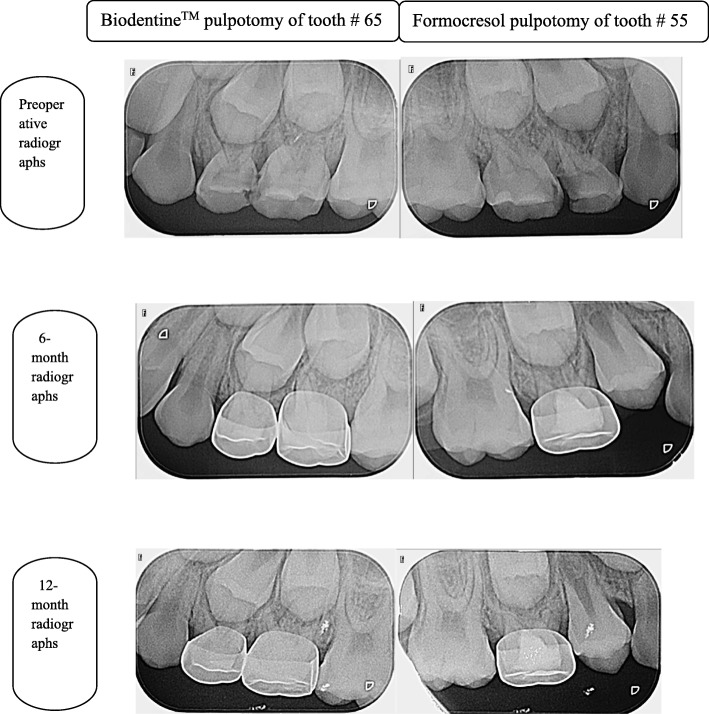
Periapical radiographs showing successful Biodentine^TM^ pulpotomy of tooth 65 and formocresol pulpotomy of tooth 55 (maxillary second primary molars)
